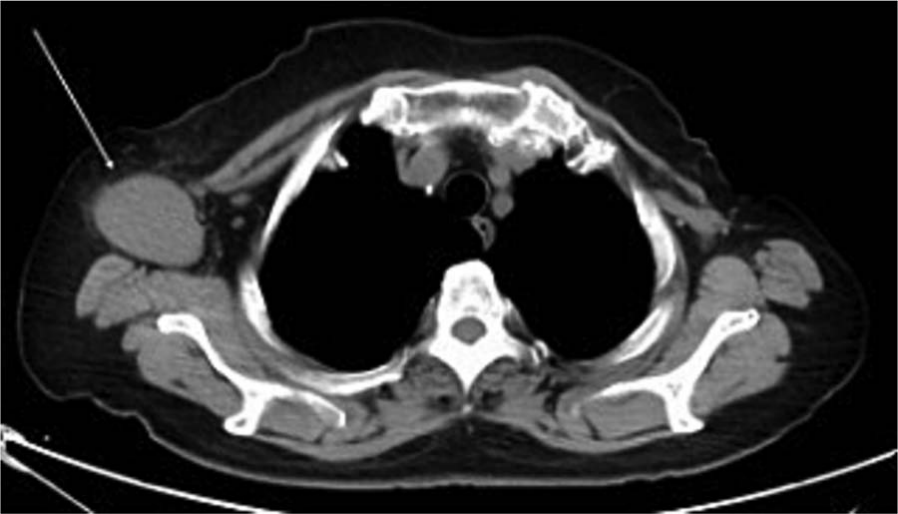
Chest computed tomography (CT) findings. A lymph node enlarged to 46 × 34 mm was observed in the right axilla.
